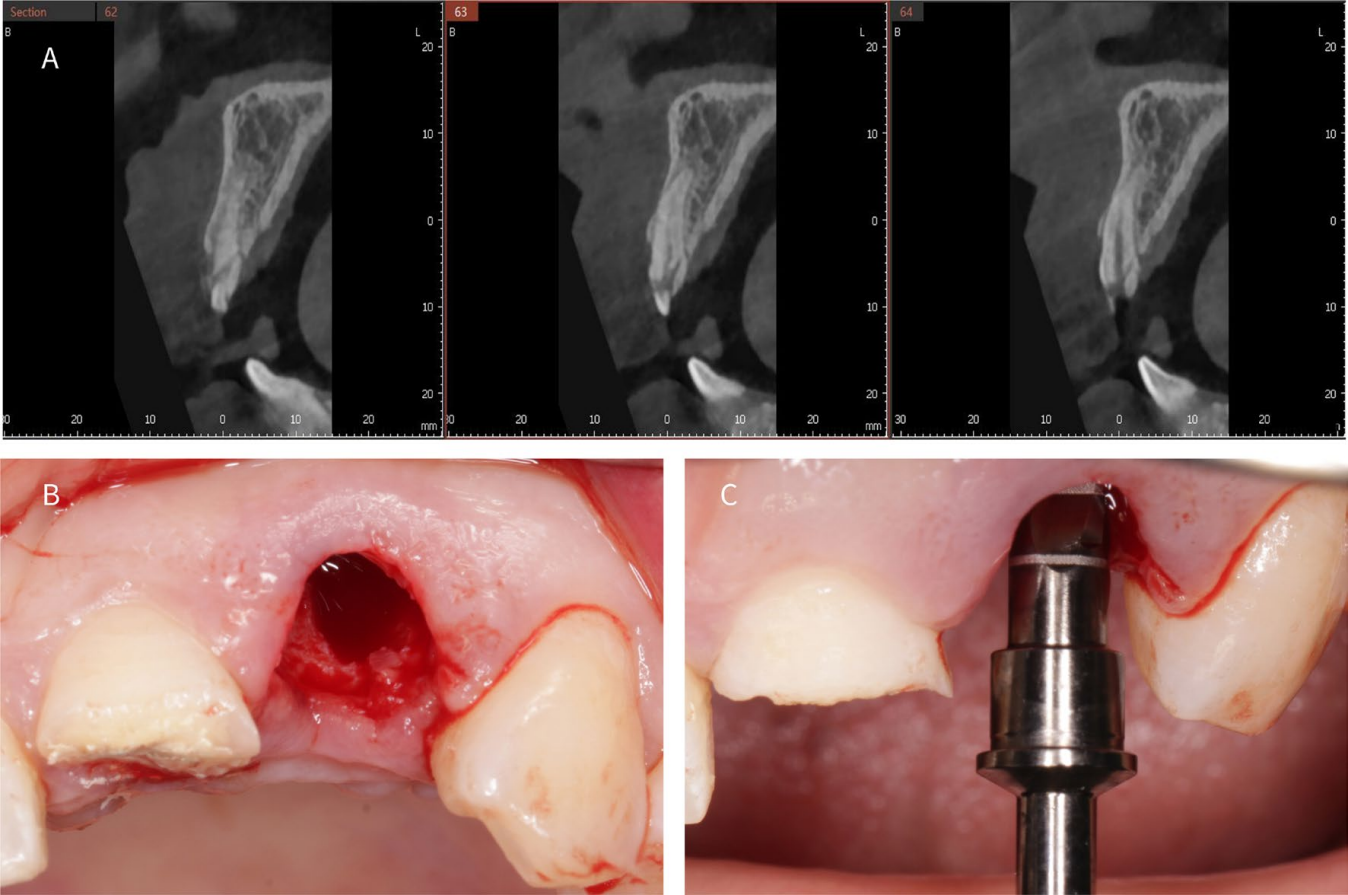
(A) Preoperative CBCT showing an intact but thin facial bone wall at tooth #22. (B) Socket‐shield preparation with the buccal root fragment retained and refined. (C) Sequential implant site preparation using the manufacturer's drilling protocol, with the final drill positioned toward the palatal aspect of the socket.
